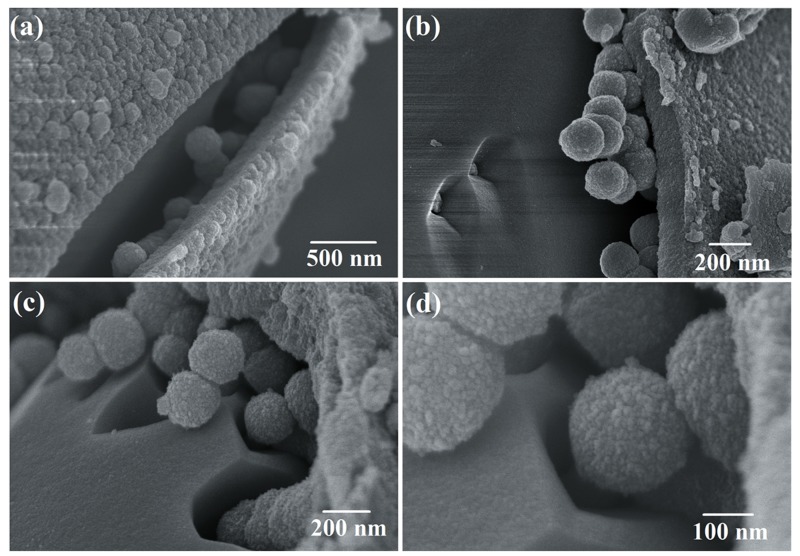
The SEM images of (a,b) S3 and (c,d) the detail of S3 treated by ultrasonic.
